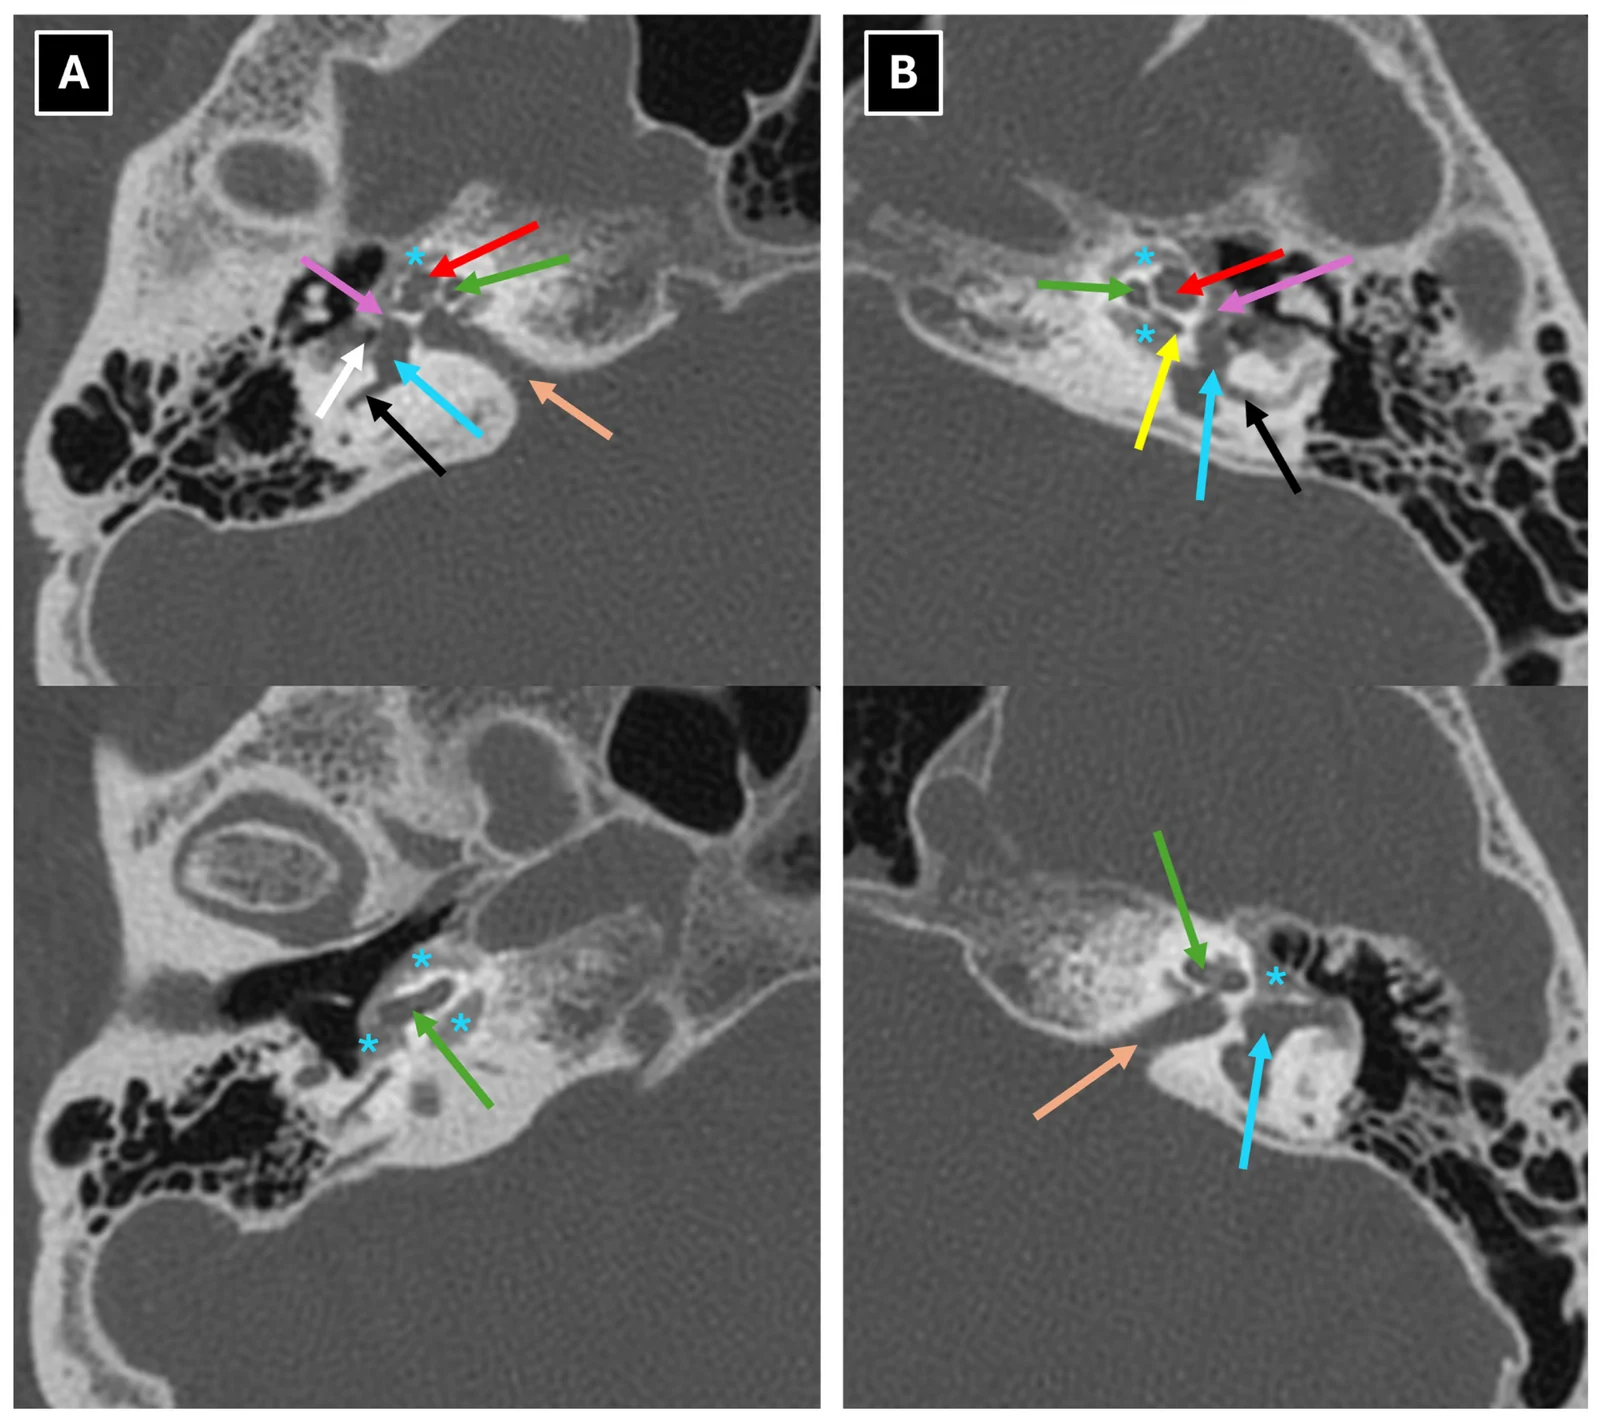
High-resolution computed tomography (CT) of a bilateral cochlear grade 3 otosclerosis in a 59-year-old woman. Axial images of the right (A) and left (B) temporal bones demonstrate extensive, relatively symmetric pericochlear hypoattenuating demineralization involving multiple cochlear turns and producing the characteristic “double-ring” appearance. Otospongiotic changes extend toward the vestibule, fissula ante fenestram, oval window, and lateral semicircular canal. The bilateral diffuse and confluent cochlear involvement corresponds to grade 3 otosclerosis according to the Symons–Fanning CT classification. An enlarged vestibule due to the otospongiotic changes can be seen. CT images were acquired with a “GE Revolution Maxima 64-slice” machine and reconstructed at 0.6 mm slice thickness. Black arrow—lateral semicircular canal; blue arrow—vestibule; blue asterisk—otosclerosis; green arrow—basal turn of cochlea; orange arrow—internal auditory canal/vestibulocochlear (VIII) nerve; pink arrow—fissula ante fenestram—the most common site of otosclerotic involvement; red arrow—middle turn of cochlea; white arrow—oval window; yellow arrow—cochlear window. The radiologic diagnosis of otosclerosis is based on high-resolution computed tomography [1]. Axial CT is considered the primary projection for the diagnosis of otosclerosis, as the relevant anatomical structures are best visualized on these images [2].
